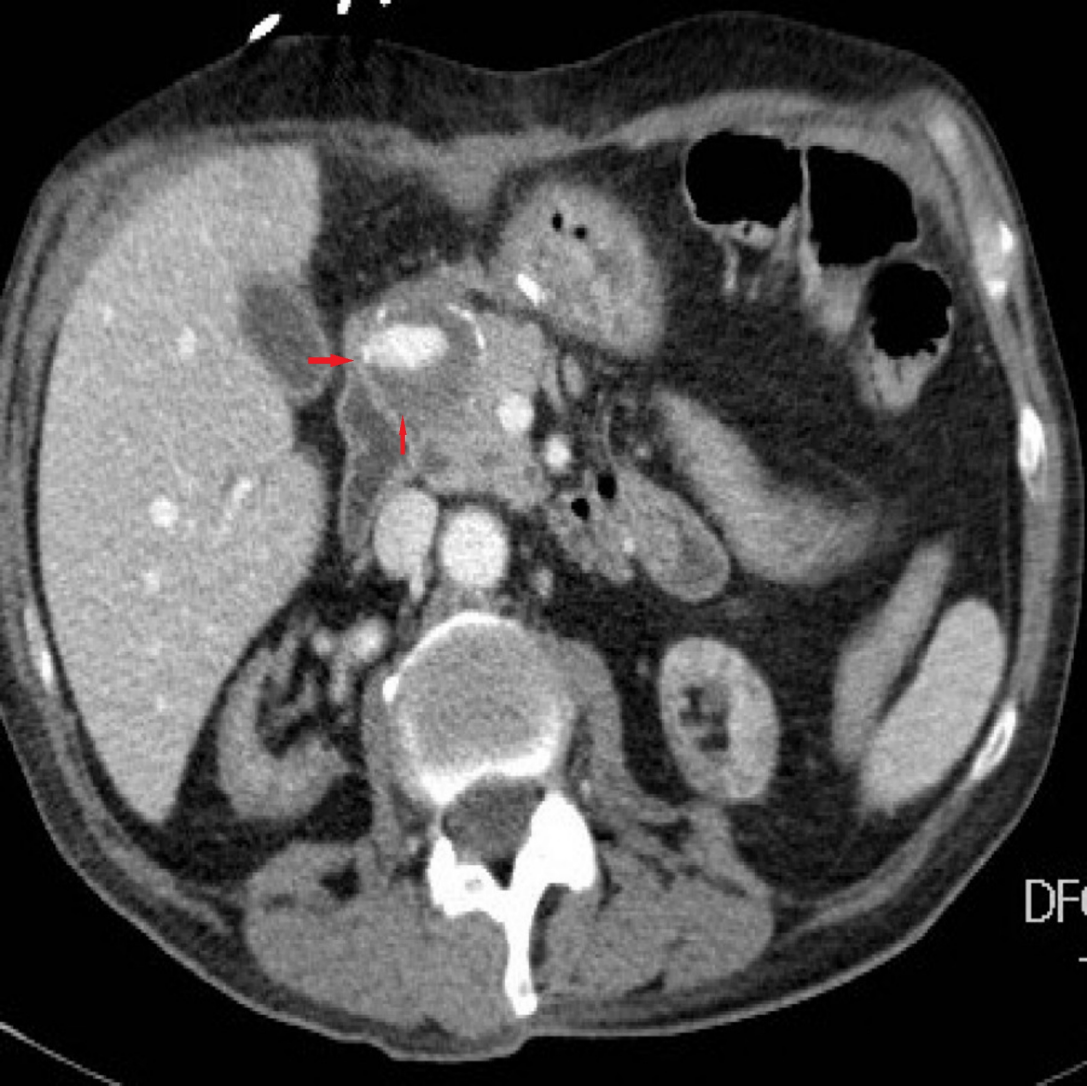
Contrast-enhanced computed tomography (axial view) showing contrast material extravasation from gastroduodenal artery pseudoaneurysm
